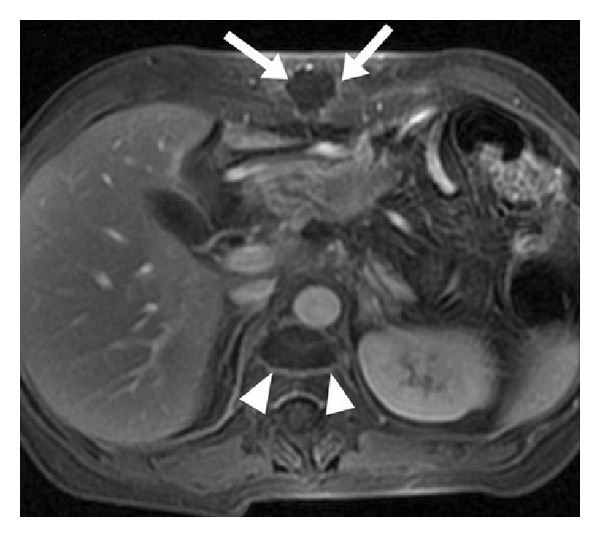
Large rim-enhancing areas of fat necrosis (arrow head) and vertebral body necrosis (arrow) along the ultrasound propagation path in a pancreatic cancer patient two weeks after HIFU ablation in fat-saturated T1-weighted magnetic resonant image after gadolinium infusion (used with permission [7]).
